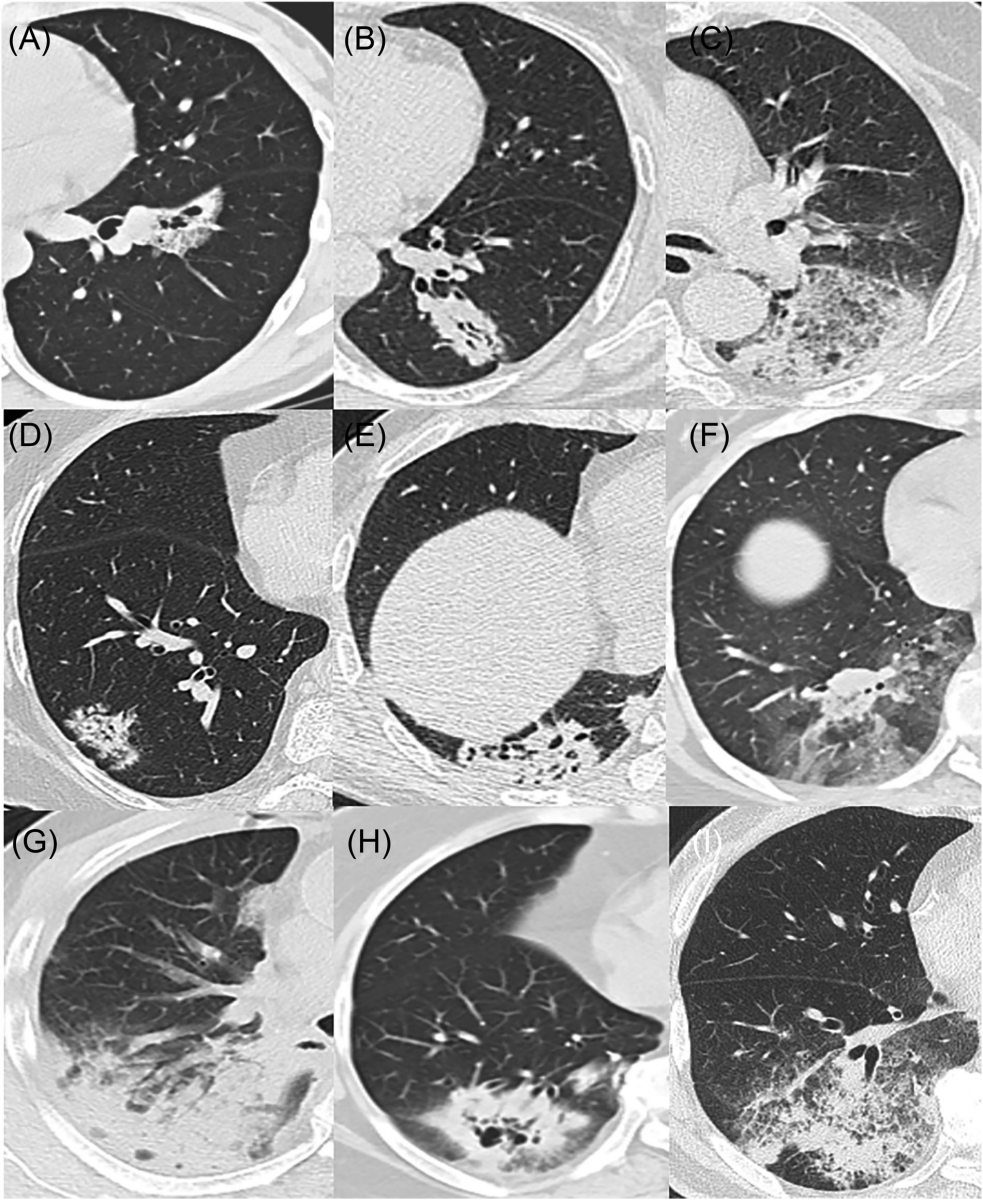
CT manifestations of pulmonary mucinous adenocarcinoma with localized pneumonia. (A) Patchy density was located in the anterior medial basal segment of the lower lobe of the left lung. (B) Patchy density was located in the posterior basal segment of the lower lobe of the left lung. (C) Patchy density was located in the dorsal segment of the lower lobe of the left lung. (D) Patchy density was located in the basal segment outside the lower lobe of the right lung. (E) Patchy density was located in the posterior basal segment of the lower lobe of the right lung. (F) Patchy density was located in the basal segment inside and posterior basal segment of the lower lobe of the right lung. (G) Patchy density was located in the posterior segment of the right upper lobe. (H) Patchy density was located in the posterior basal segment of the right lower lobe. (I) Patchy density was located in the lateral basal segment and posterior basal segment of the right lower lobe.
